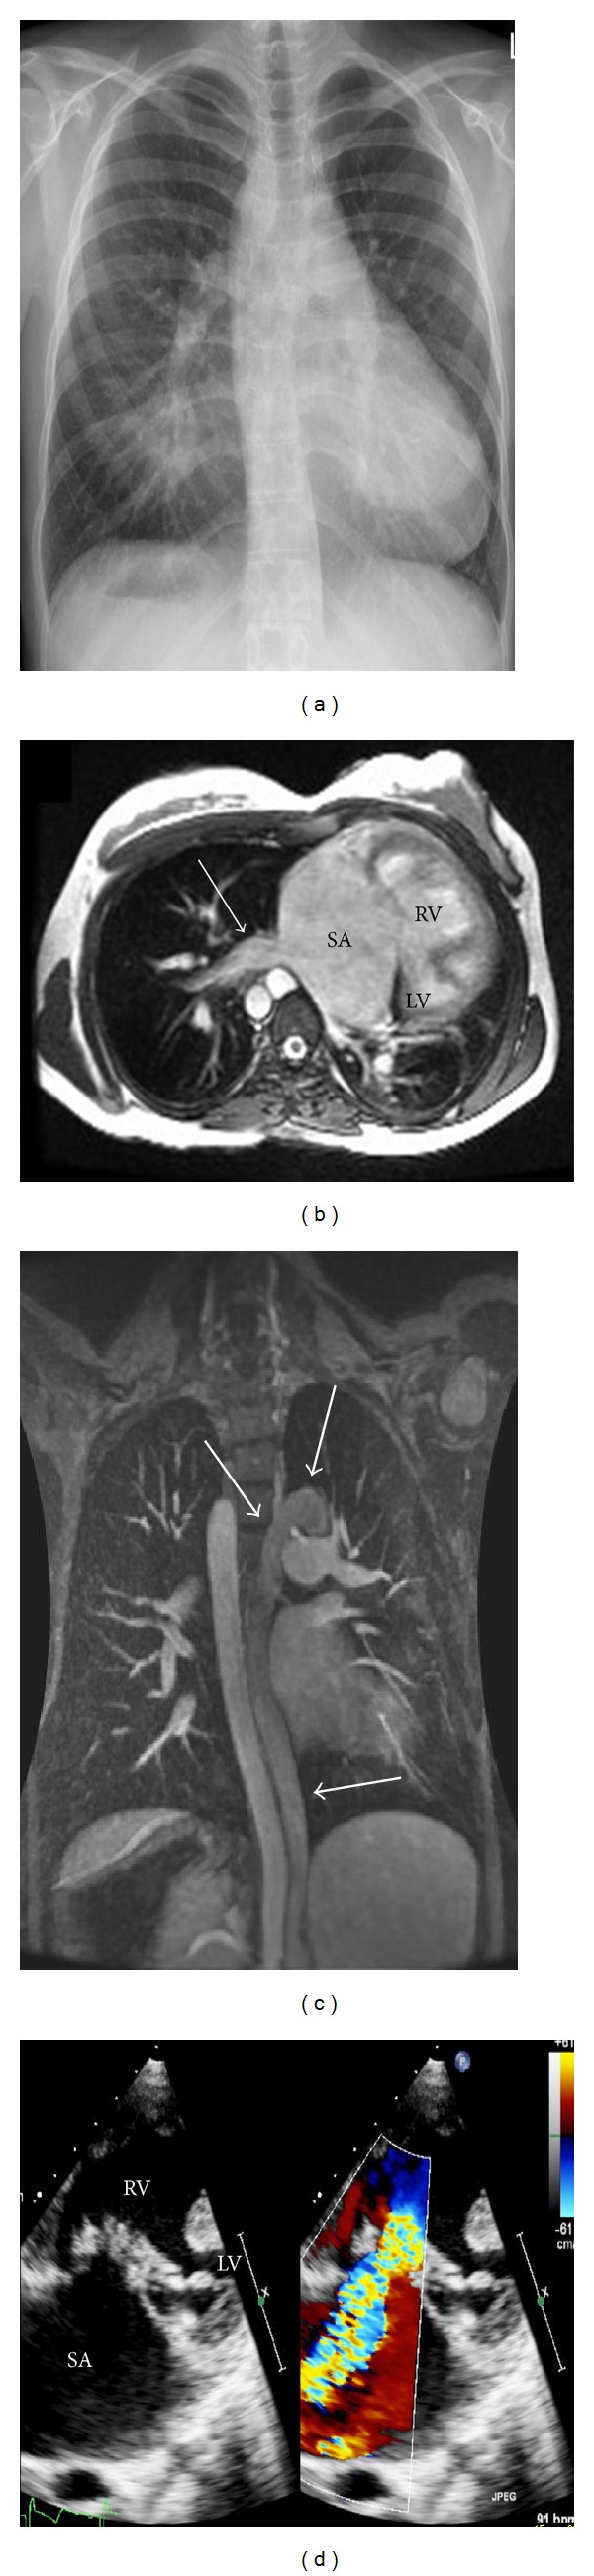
24 year old patient with heart failure and complex cardiac anatomy in the setting of left atrial isomerism. (a): Chest X-ray. (b): Axial slice of cardiac magnetic resonance imaging, showing a single atrium (SA) with direct connection of hepatic veins (arrow), dominant right ventricle (RV) and hypoplastic left ventricle (LV). (c): Coronal slice of cardiac magnetic resonance imaging showing hemiazygous continuation to left superior caval vein (arrows) in the absence of a right superior caval vein. (d): Apical echocardiographic view demonstrating the dominant right ventricle (RV), the hypoplastic left ventricle (LV), the enlarged single atrium (SA) and the dysplastic tricuspid valve. On the right hand of the panel color Doppler demonstrates severe tricuspid regurgitation.
